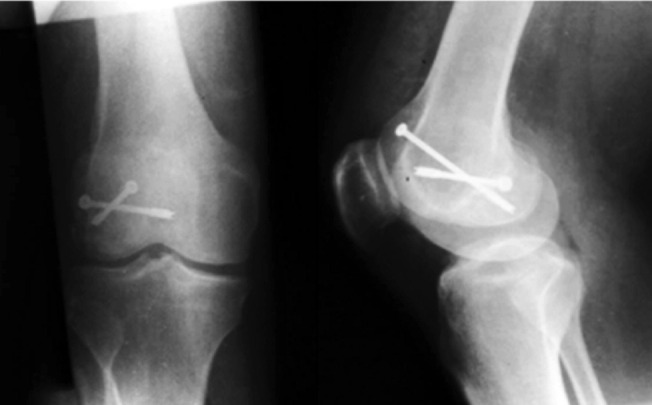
Radiographs at 12 months follow-up showing fixation of fracture with two screws.
